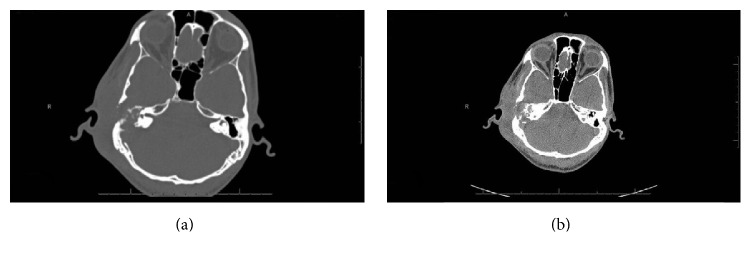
Axial CT scan (noncontrast) showing the temporal bone. (a) Bone window and right ill-defined soft tissue density in the right mastoid air cells, middle, and inner ear, associated with bony destruction. Only the basal turn of the cochlea and part of the vestibule are visualized. (b) Soft tissue window and posterior aspect of the tegmentum tympani appear destroyed, thinned, and interrupted, with subcutaneous soft tissue swelling adjacent to the EAC and collection at the superior aspect.
